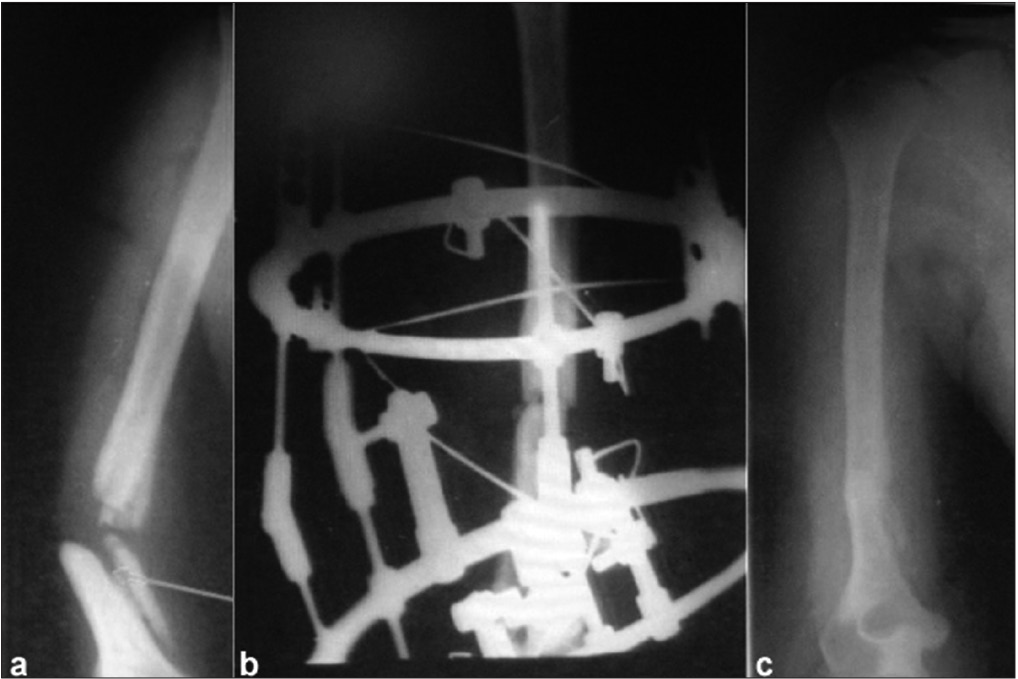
Anteroposterior radiograph of a 31 year old patient showing (a) 9 months old aseptic nonunion after conservative treatment (b) ring fixator *in situ* (c) Radiological union at 24 weeks
